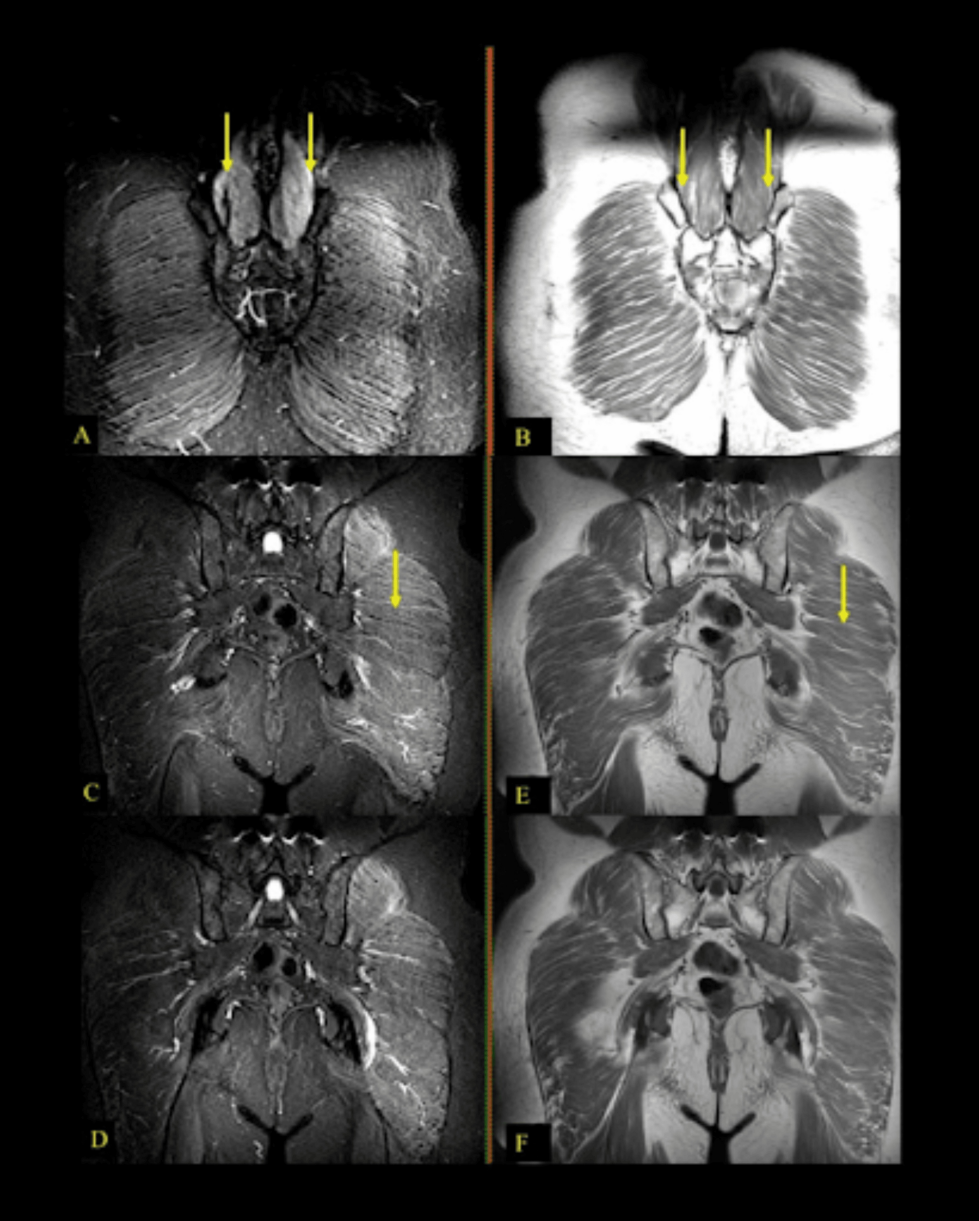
Pelvic MRI findings demonstrating bilateral paravertebral muscle atrophy with edema and partial fatty replacement (A-B: coronal STIR and T1 sequences, arrows). Bilateral gluteal muscle atrophy, more pronounced on the left, with edema and partial fatty replacement (C-F: coronal STIR and T1 sequences).
